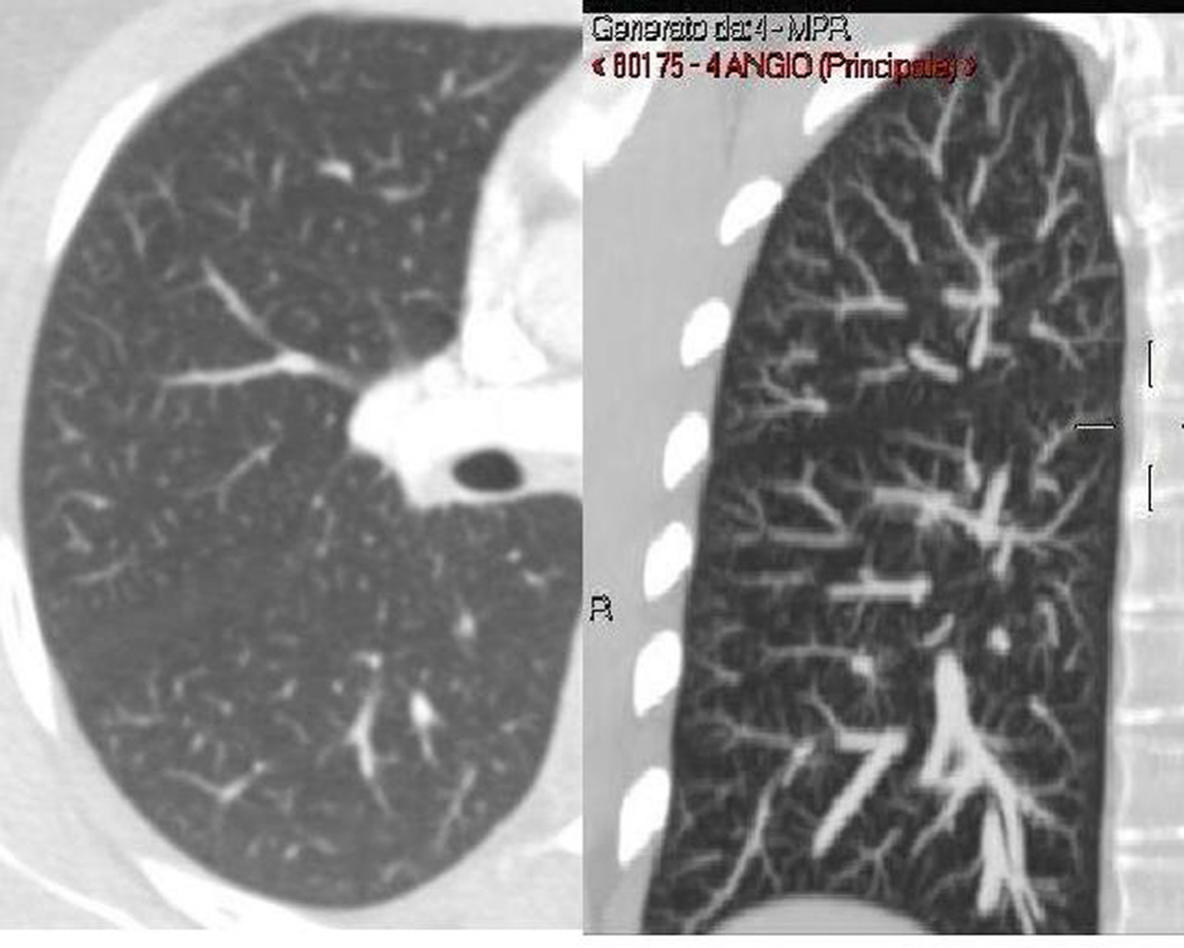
Sliding thin-slab MIP image obtained from single section CT angiographic data (section collimation 64x0.6 mm; slice thickness 2 mm; slice increment 1 mm; rotation time 0.5 sec) reveals centrilobular vessel–associated micronodules connected by arcade-like dilated subpleural vascular branches.
